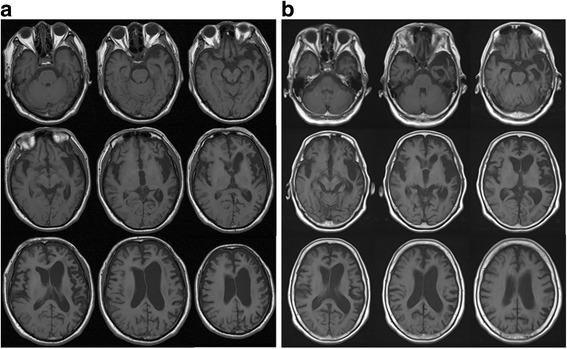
Brain MRI (axial T1-weighted images) of the patient’s brother (a) and the patient (b). a T1-weighted brain images of the patient’s brother at 4 years after disease onset. Atrophy was predominantly observed in the left hemisphere affecting the frontotemporal lobes. b T1-weighted brain images of the patient at 1 year after disease onset. Similar to her brother, atrophy was predominantly observed in the left hemisphere affecting the frontal and temporal lobes
